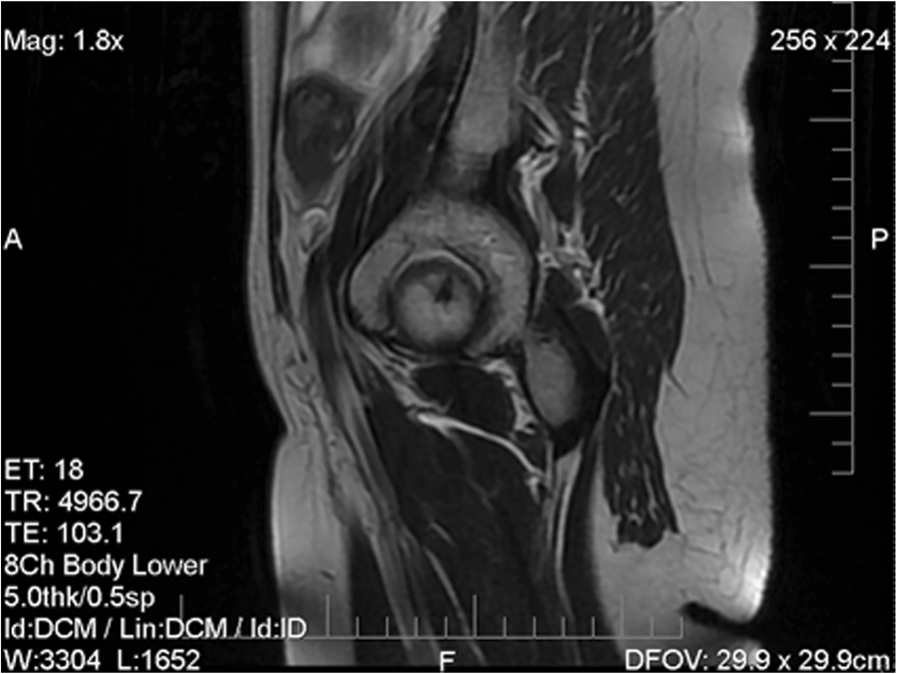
Magnetic resonance imaging of the pelvis: sagittal view. An enhancing mass is visible in the anterior cervix
